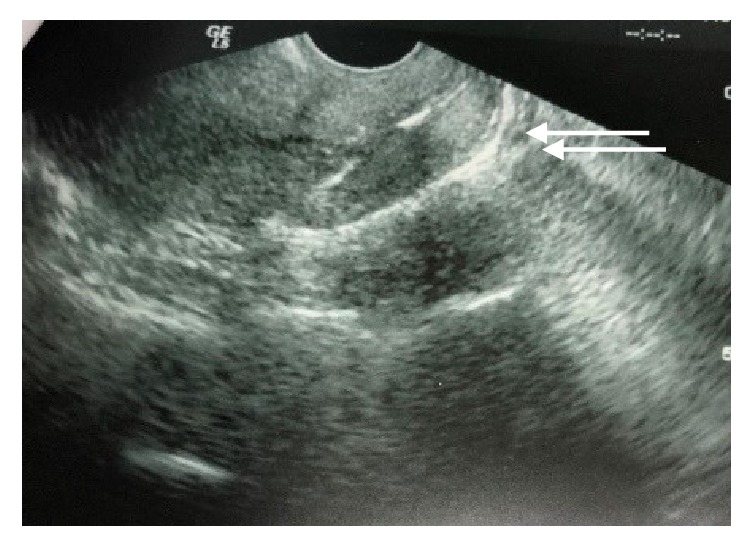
Ultrasound showing a hyperechoic cervix.
